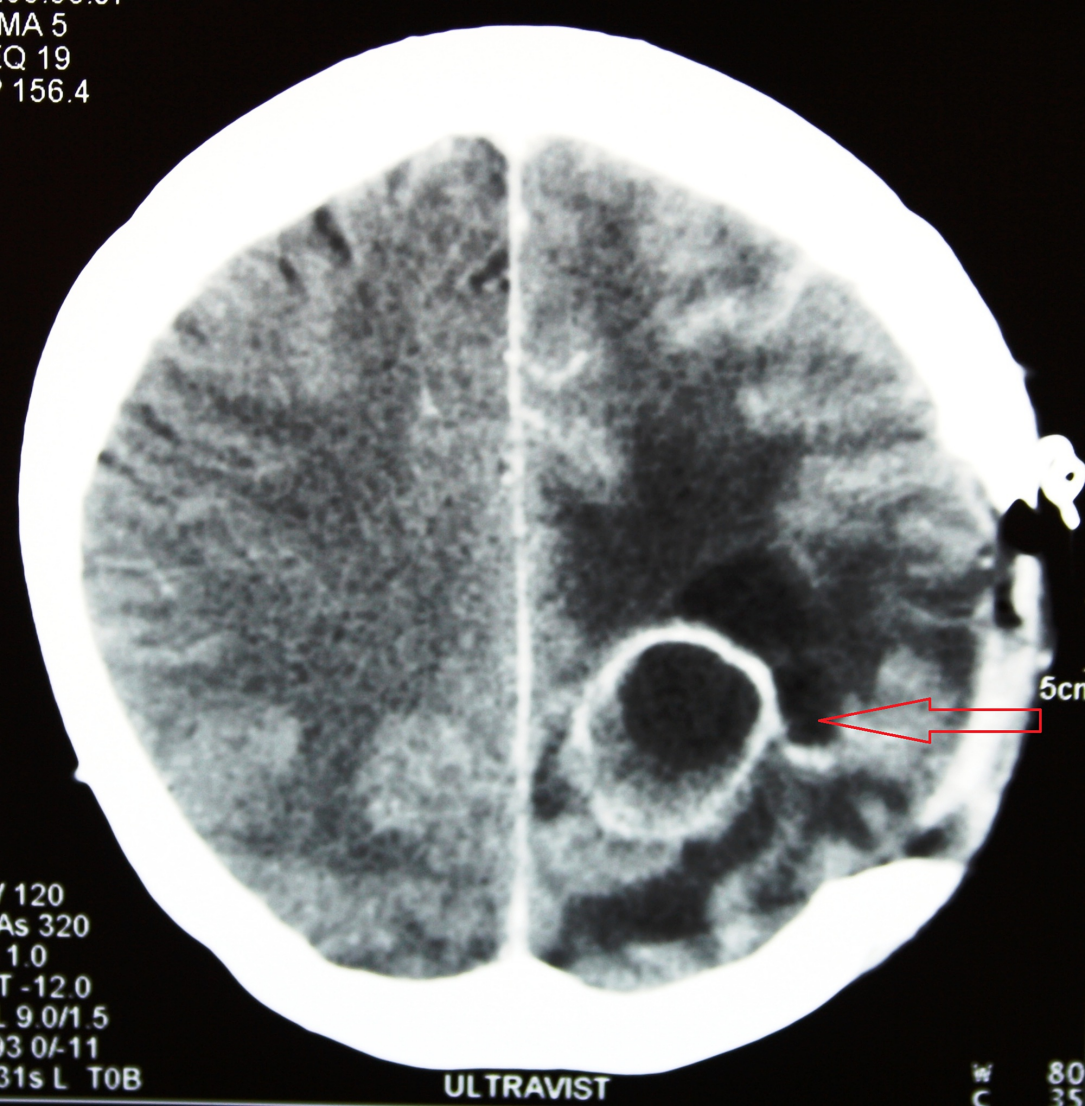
A CT scan obtained 3 weeks after first re-surgery revealed a new incapsulated process in the proximity of the one removed during the first surgical procedure.
